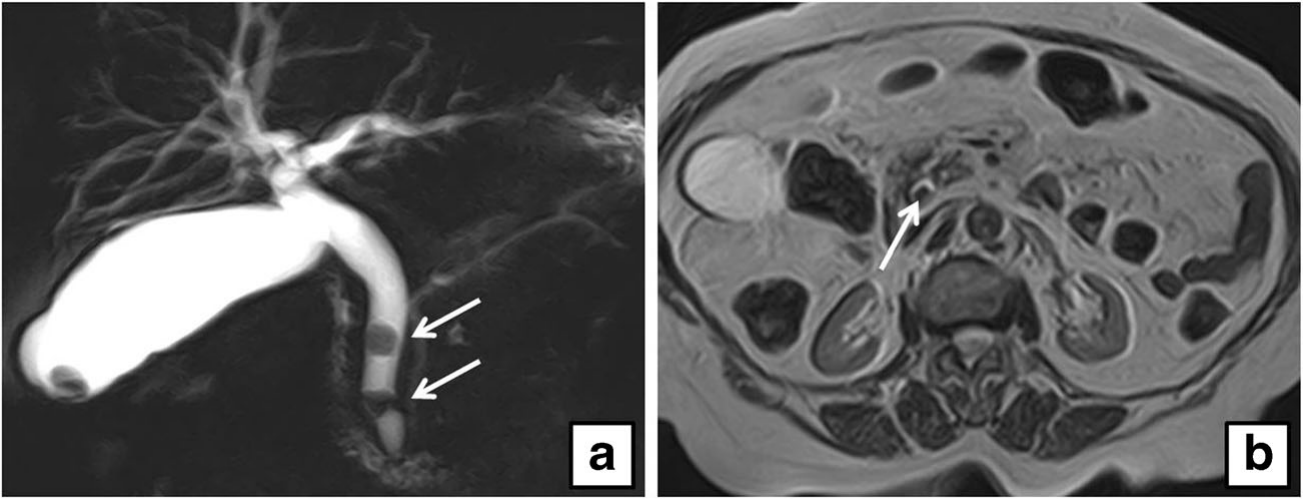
a Coronal maximum intensity projection (MIP) reformat shows two filling defects in a dilated CBD (*arrows*) and one in the gallbladder. b Axial T2-weighted MRI of the same patient shows a filling defect in a dilated CBD (*arrow*)
